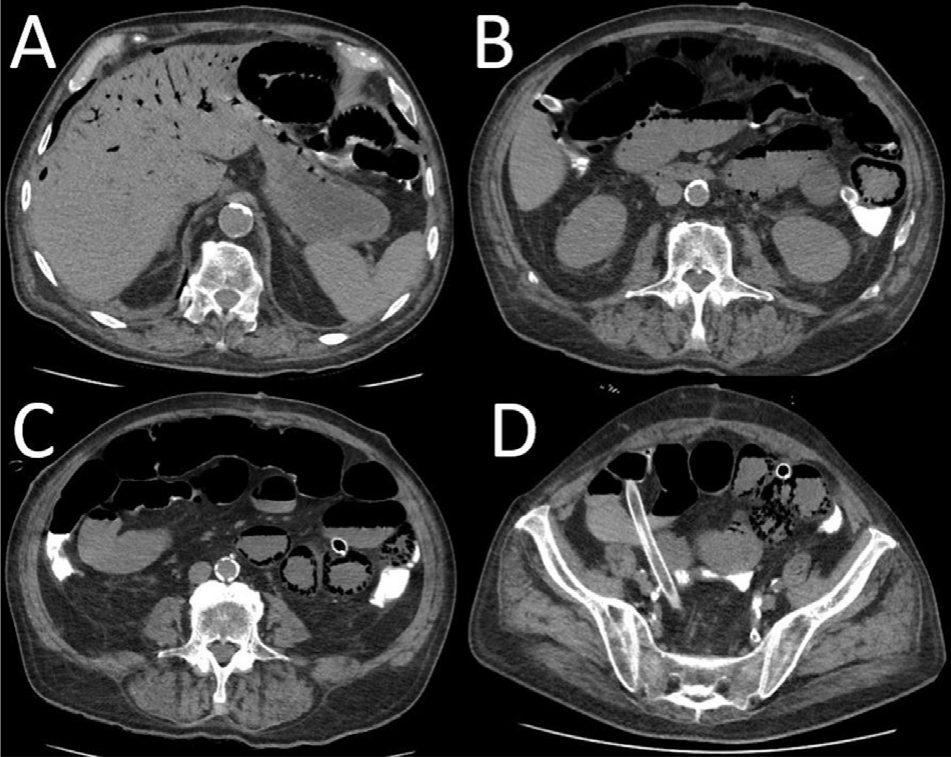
Oral contrast CT showing pneumatosis intestinalis (B-D) in different gut tracts and pneumobilia within intrahepatic biliary tree (A).
